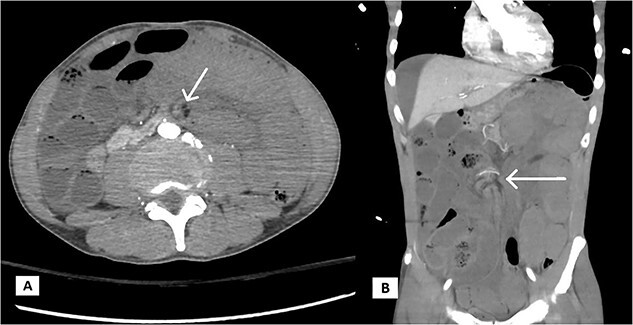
Computed tomography angiography of the abdomen with areas of “swirls” (white arrows) concerning for volvulus. A, axial and B, coronal views.
